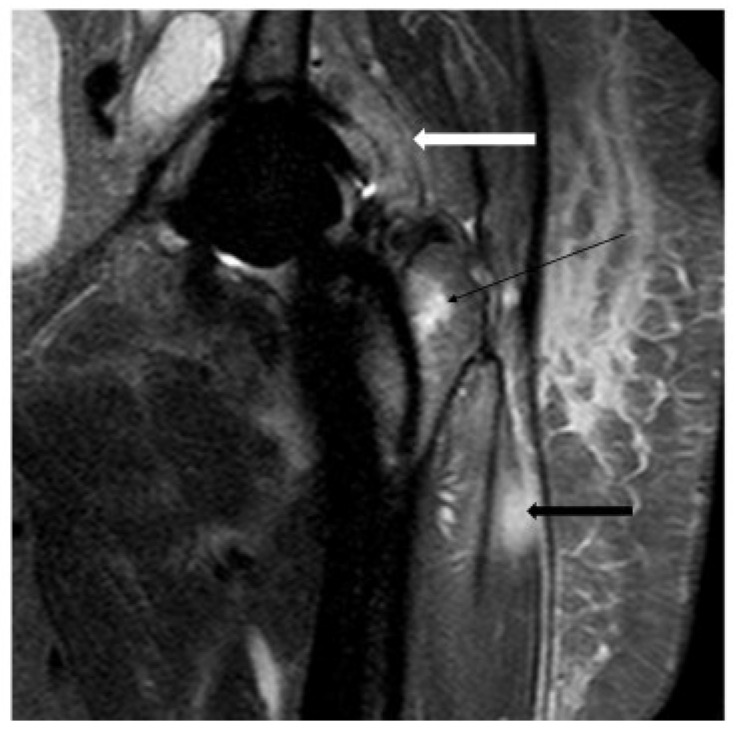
MRI of PJI after hip arthroplasty; MRI imaging reveals layering (white arrow) and synovial hyperintensity, indicating an infection. Femoral bone marrow (a black, thin arrow) and muscle edema (black thick arrow) suggest periprosthetic stress reaction [80].
